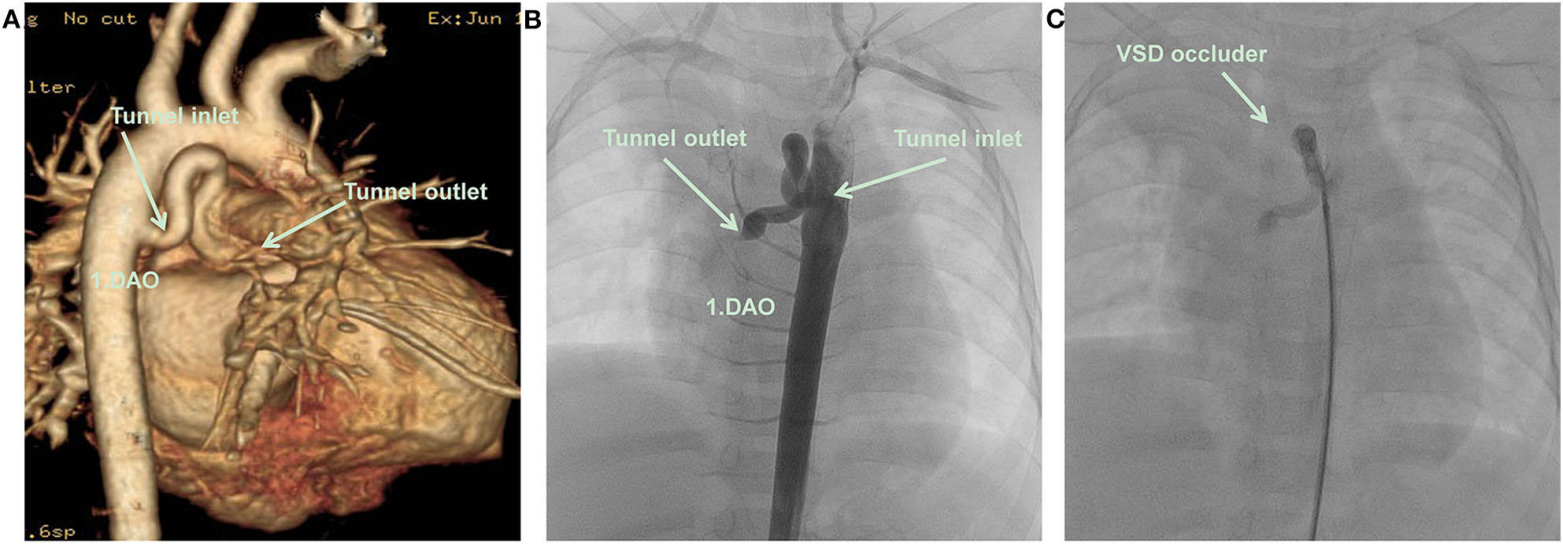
(A) CTA: An abnormal blood vessel developed from descending aorta, and zigzagged to the right atrium. (B) Cardiac catheterization: An abnormal blood vessel developed from descending aorta, and zigzagged to the right atrium. (C) A VSD occluder has been placed at the inlet of tunnel, there was no obvious shunt revealed by aortography after closure. DAO, descending aorta; RA, right atrium; VSD occluder, ventricular septal defect occluder.
